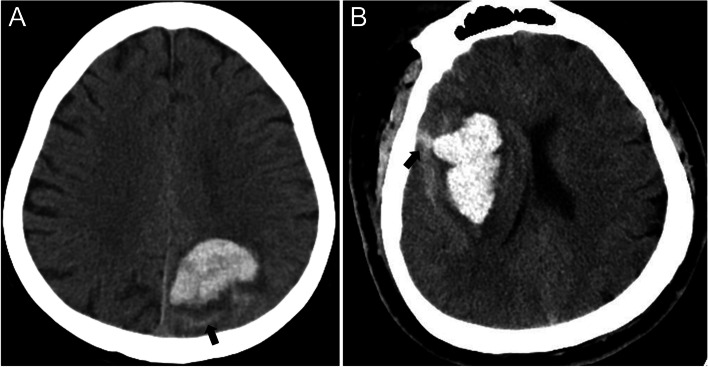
Intracerebral hemorrhage (ICH) with subarachnoid extension on baseline computed tomography. A Lobar ICH with subarachnoid extension (arrow). B Deep ICH with subarachnoid extension (arrow)
